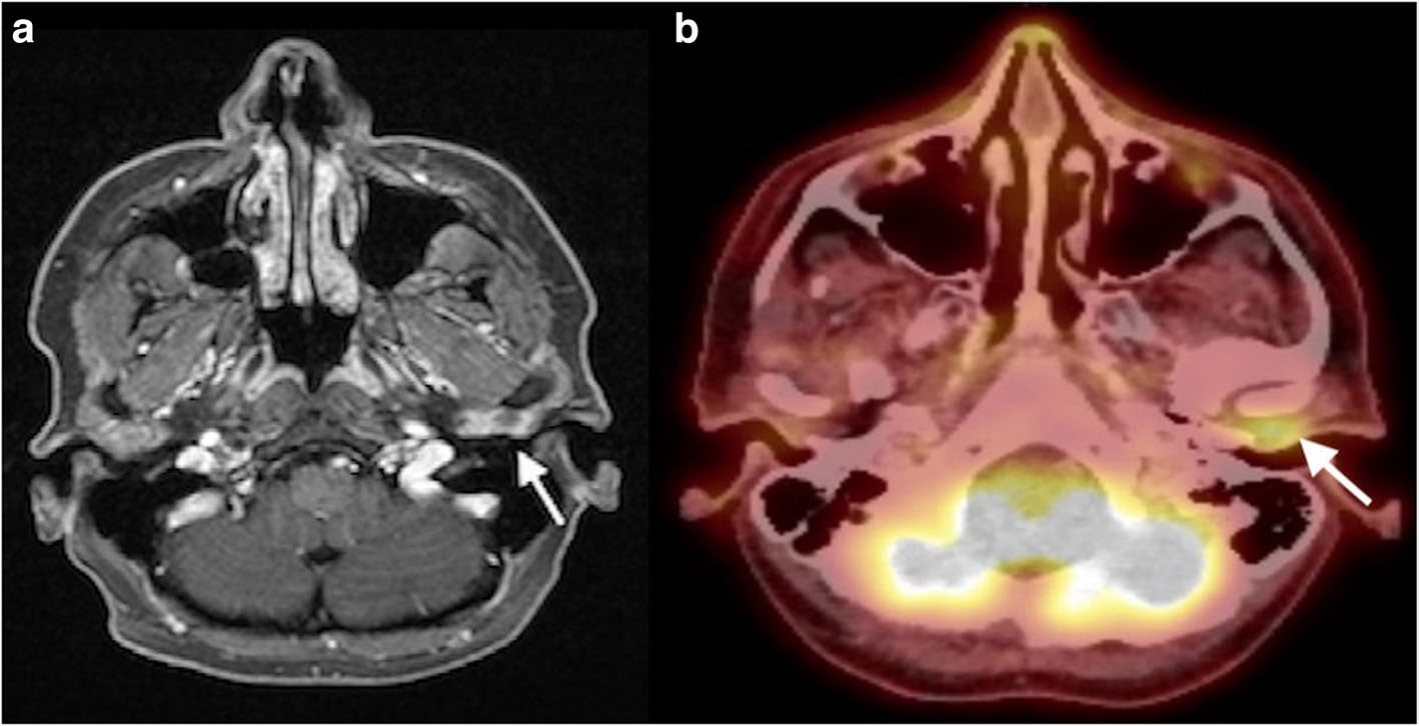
A The preoperative T2 weighted axial magnetic resonance image showing a pathological enhancement (13 × 8 × 10 mm) between the anterior ear canal wall and temporomandibular joint (arrow), (left image). B The preoperative PET/CT axial scan showing a small FDG enhanced area (arrow) in the anterior wall of the left external auditory canal (right image)
